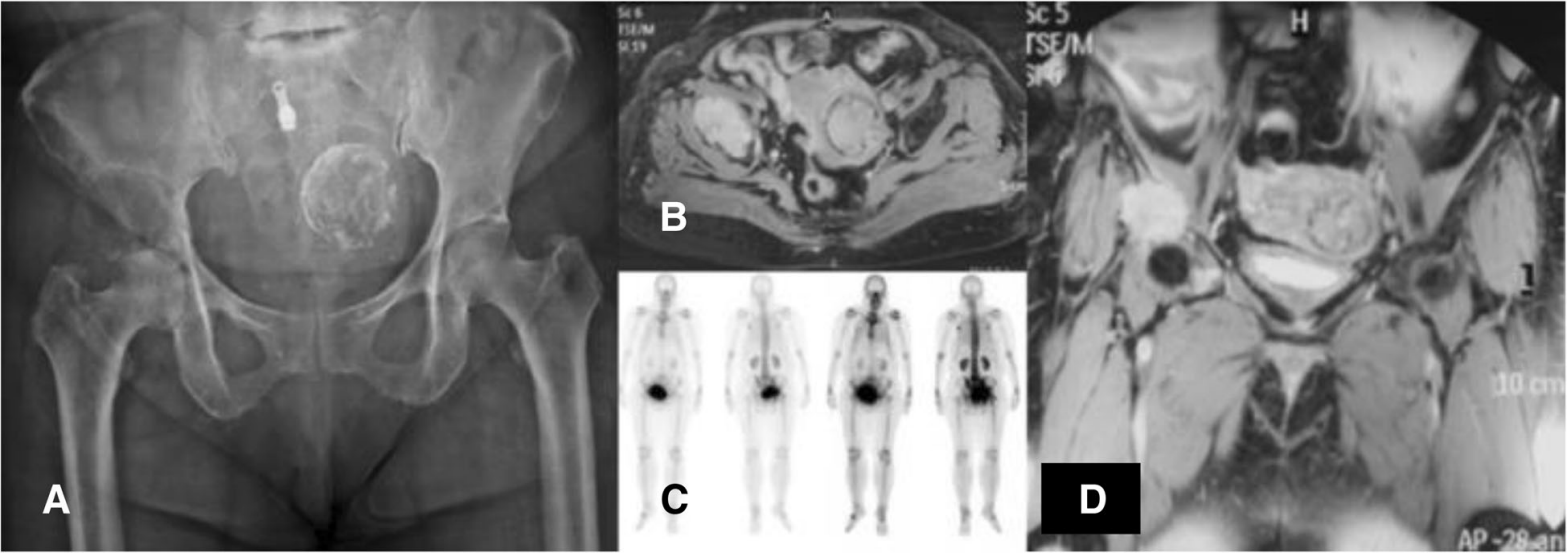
a Film images of both hips AP showing osteolytic lesion in the supra-acetabular area, (b) axial MRI revealing lesion in the right hip with soft tissue extension, (c) bone scan revealing increased uptake on right acetabulum, (d) coronal MRI showing lesion on the supra-acetabular area with cortical breakthrough
